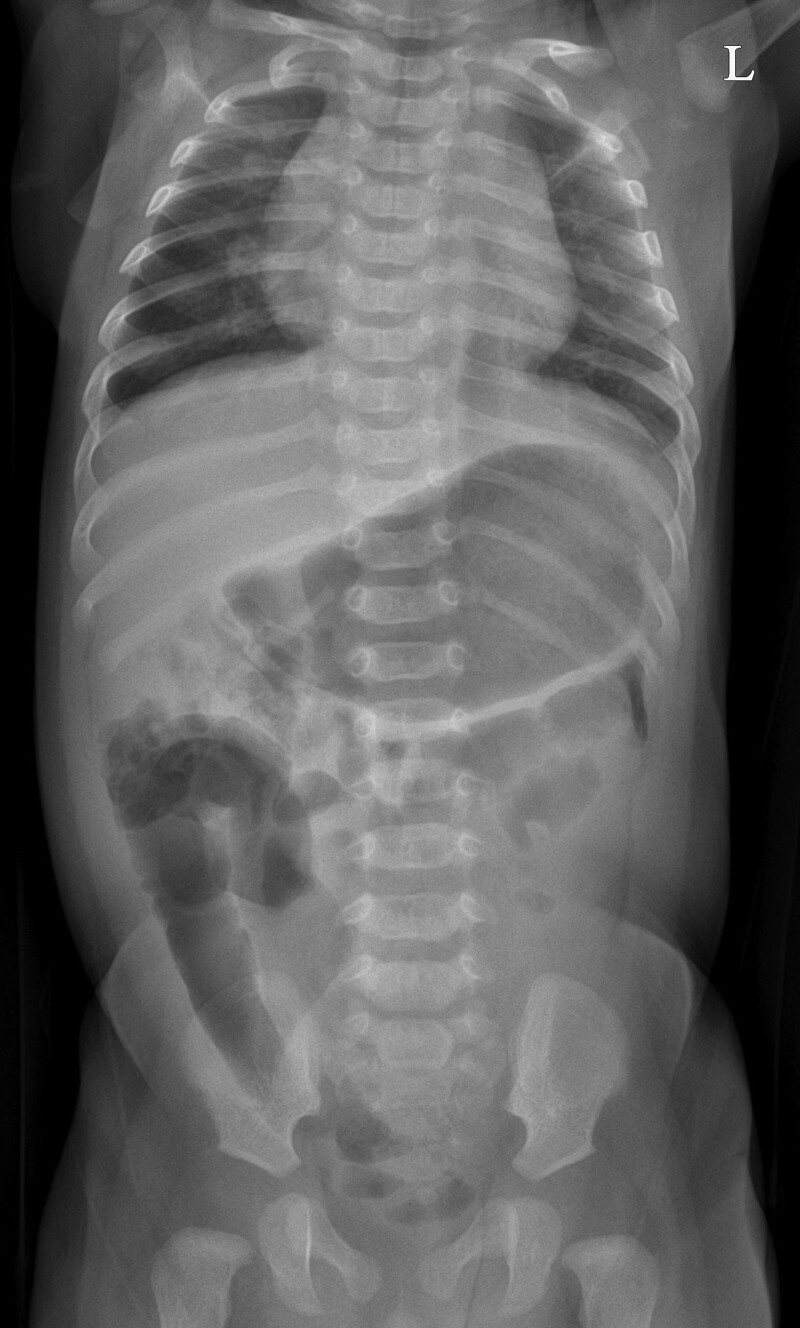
Initial X-ray of the abdomen showed no abnormalities.
